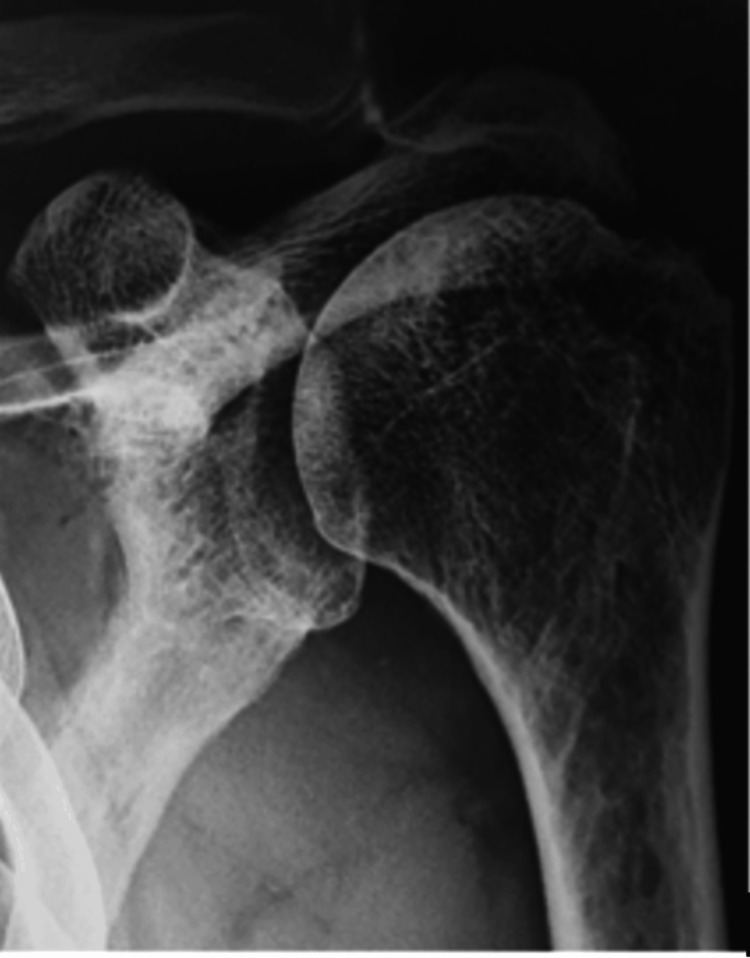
Anteroposterior radiograph of the left shoulder Superior migration of the humeral head is observed, with reduction of the acromiohumeral space to 6 mm, a finding consistent with a substantial rotator cuff tear
